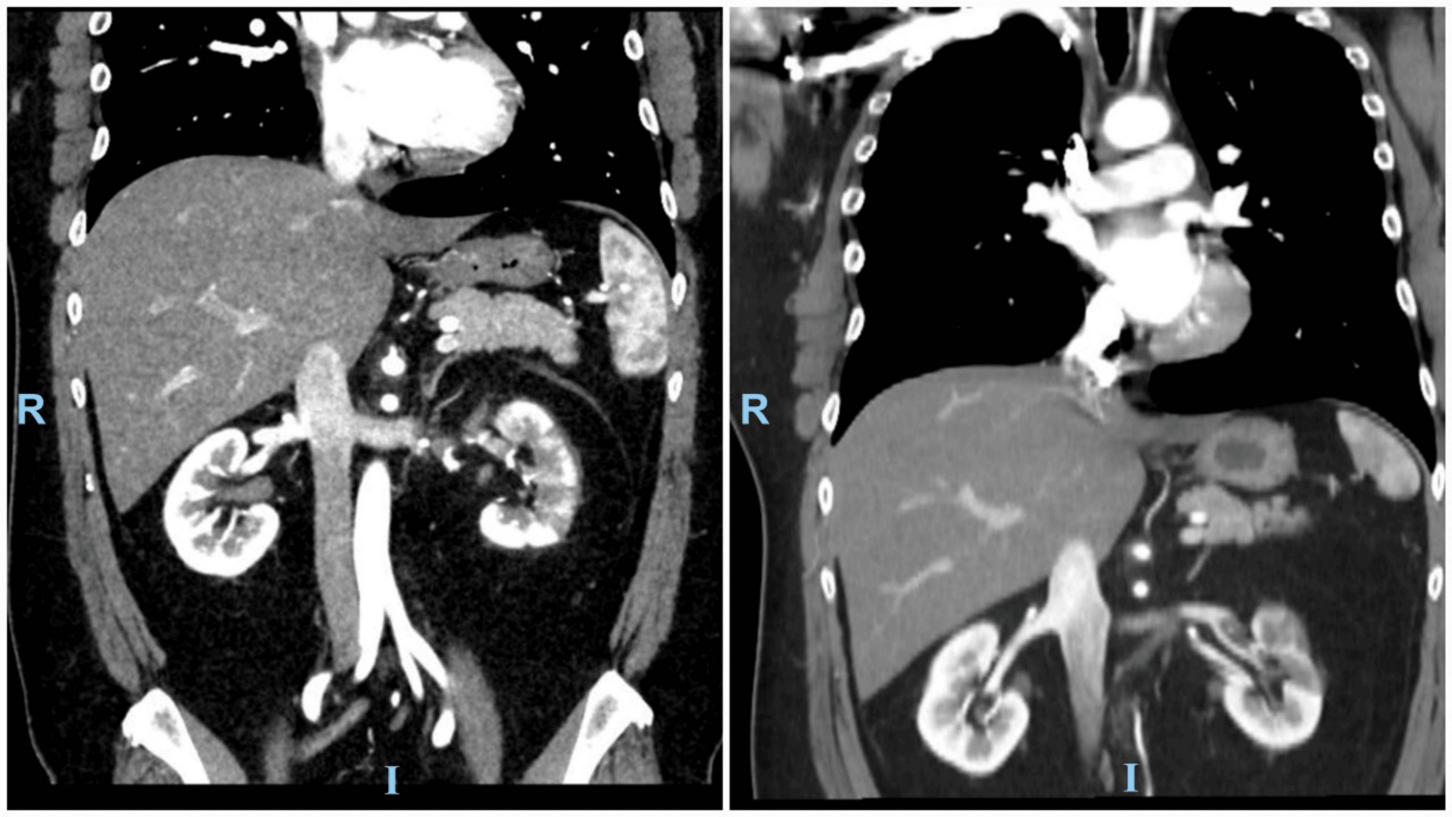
Follow-up computed tomography angiography of the aortoiliac after three weeks showing persistent filling defect with better recanalization of the left renal artery Left image: computed tomography scan upon presentation. Right image: computed tomography scan after three weeks
